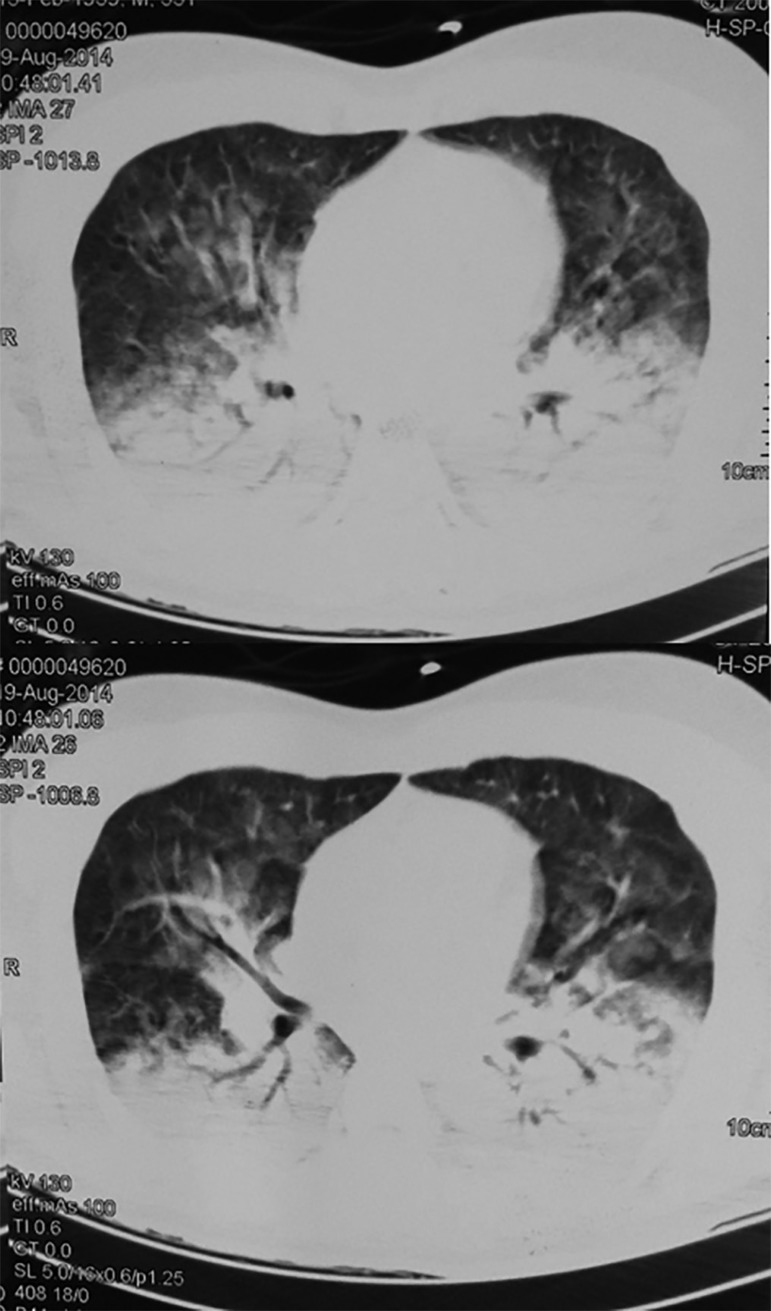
Initial chest computed tomography.
